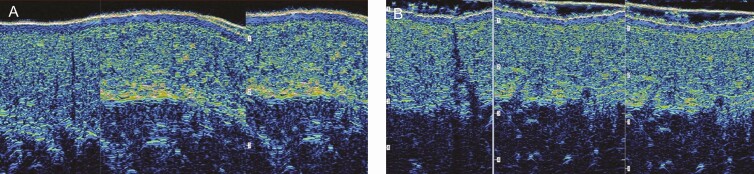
Ultrasound images of an abdominoplasty 3 to 6 weeks postoperative, demonstrating decreased fibrous banding on the R&R side. (A) bland moisturizer and (B) R&R (ReFORM & RePAIR with TriHex Technology [Alastin Skincare, Inc., Carlsbad, CA]).
